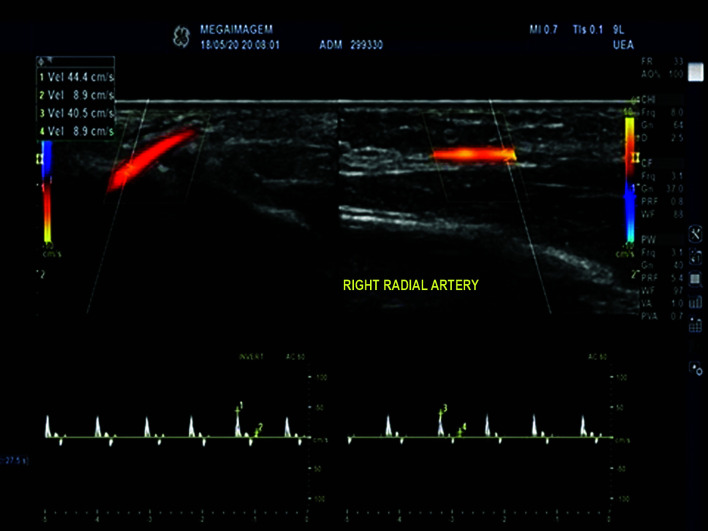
Arterial Doppler showing reduced velocity and amplitude of flow waves in the right radial artery of the wrist.
